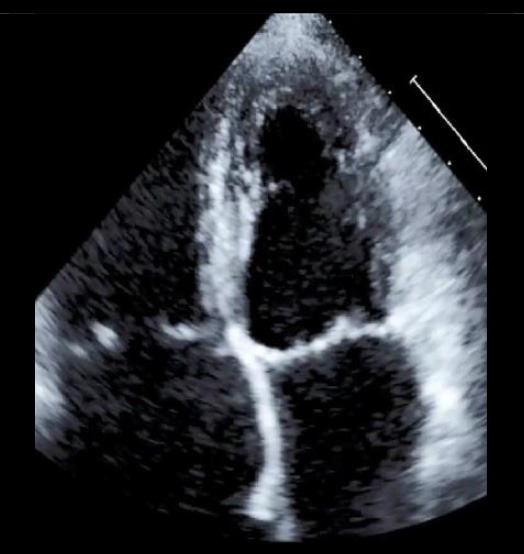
Term explanation: FTR, functional tricuspid regurgitation; ITR, isolated tricuspid regurgitation; LH-TR, left heart related tricuspid regurgitation; MRI, magnet resonance imaging; PH-TR, precapillary pulmonary hypertension related tricuspid regurgitation; RA, right atrial; RV, right ventricular; RVD, right ventricular disease related tricuspid regurgitation*.
